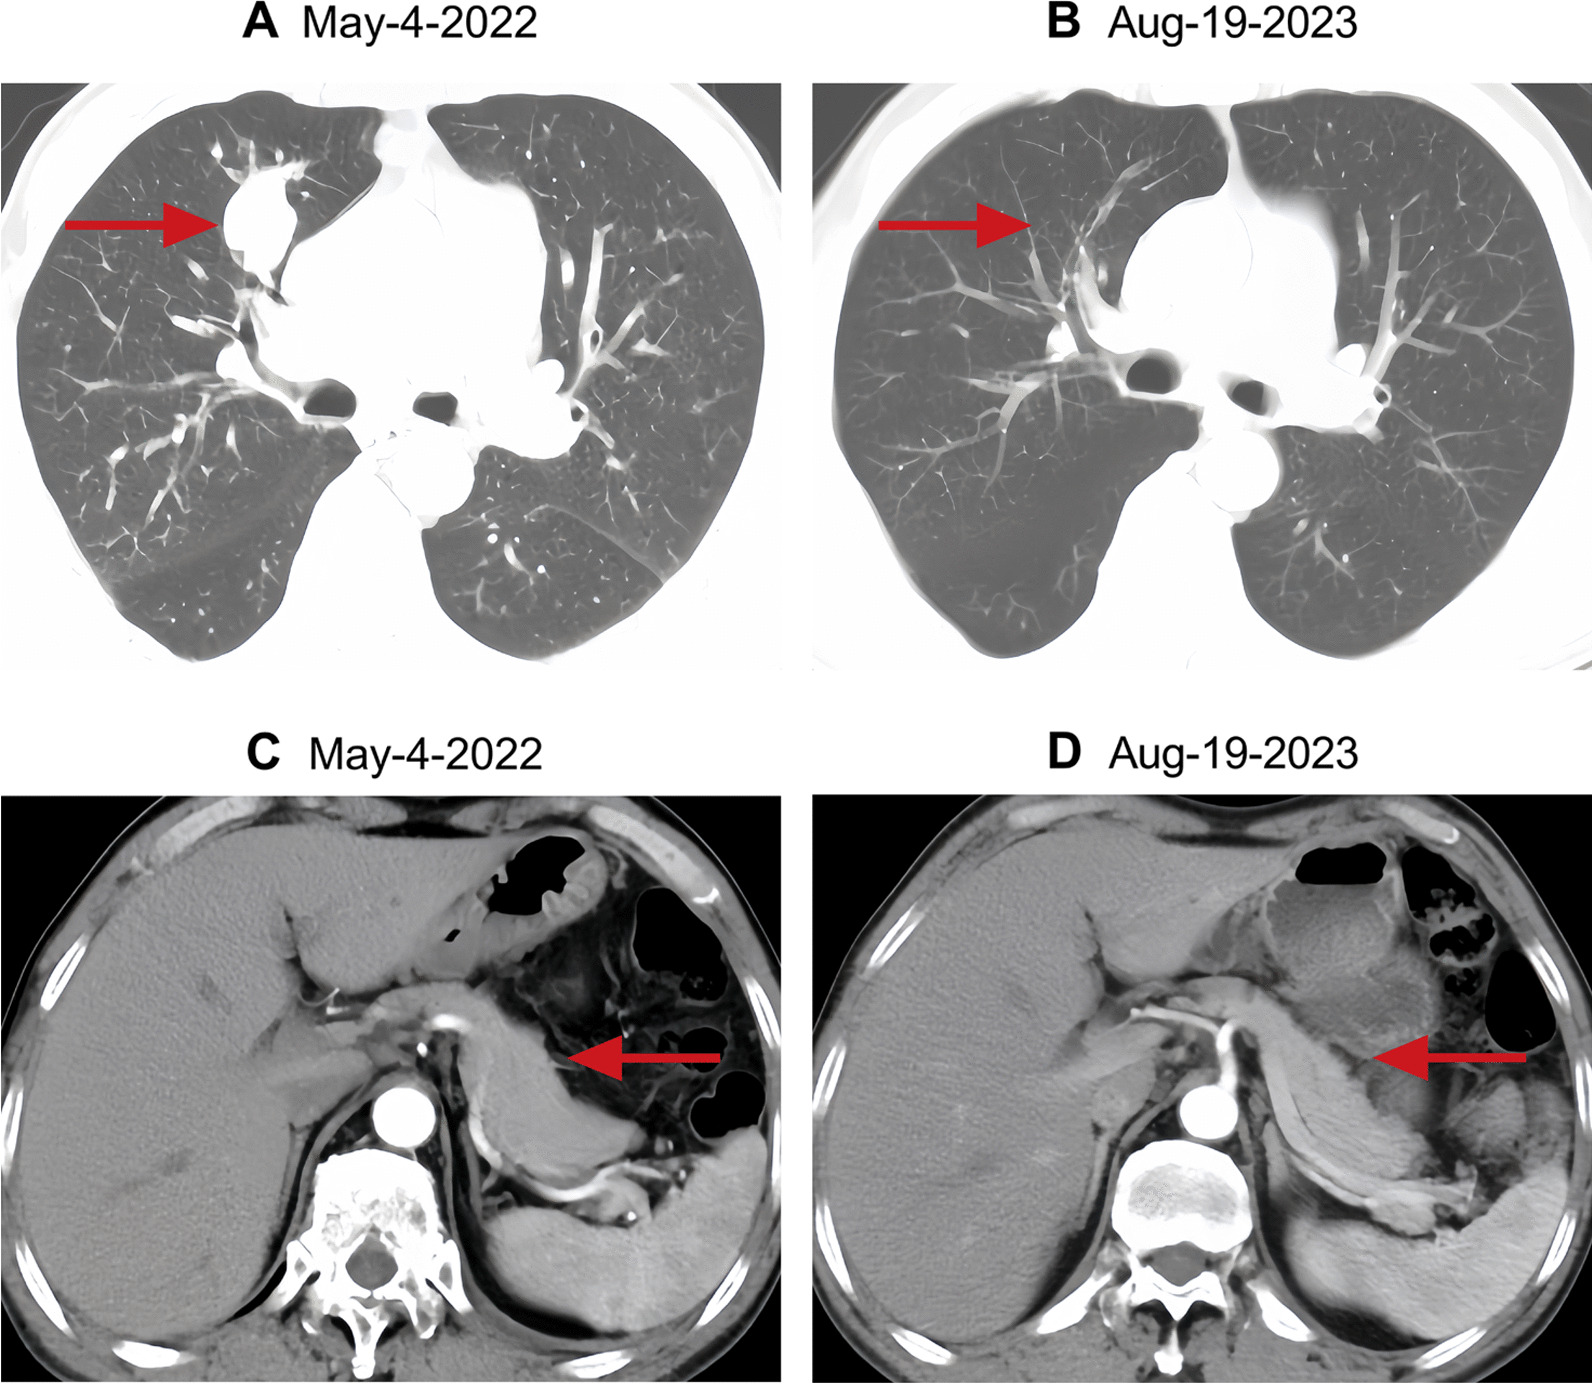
Computed tomography (CT) of the patient’s chest and abdomen during treatment. A Chest computed tomography imaging findings at the beginning of treatment. B Computed tomography of the chest of the patient with ICI-induced type 1 diabetes mellitus. Tumor lesions in B are significantly reduced versus those in A, as indicated by the arrows. C Abdomen computed tomography imaging findings at the beginning of treatment. D Computed tomography of the abdomen of the patient with ICI-induced type 1 diabetes mellitus. The pancreas in D does not show significant atrophy versus that in C, as indicated by the arrows
